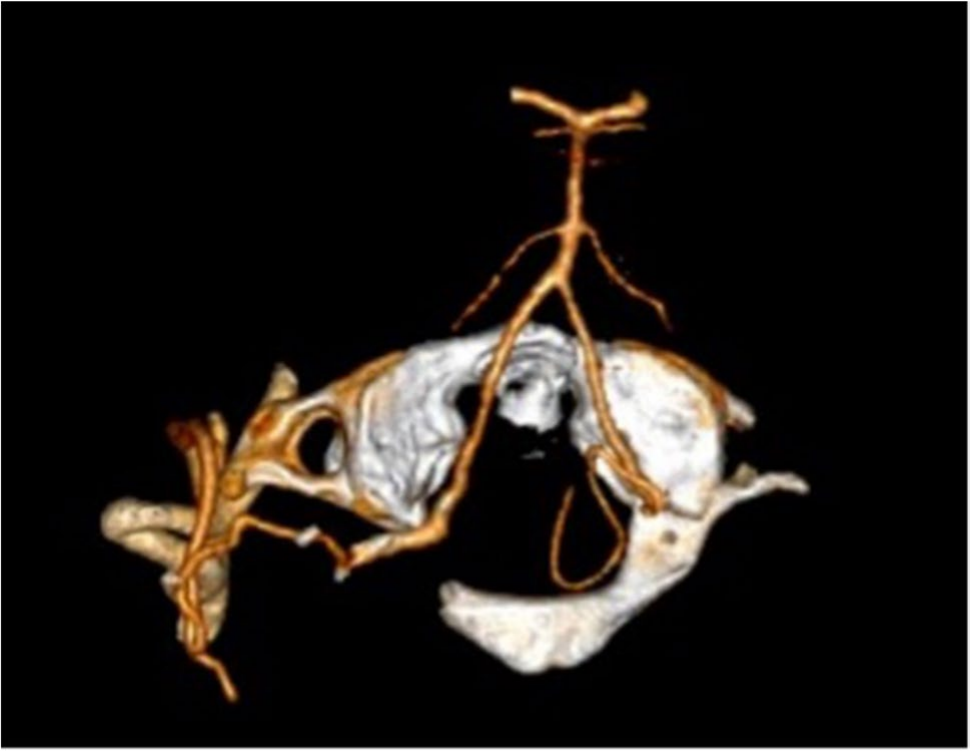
Postoperative CT angiography showing good patency of the bypass and partial removal of the posterior arch of C1 in order to expose the recipient artery
